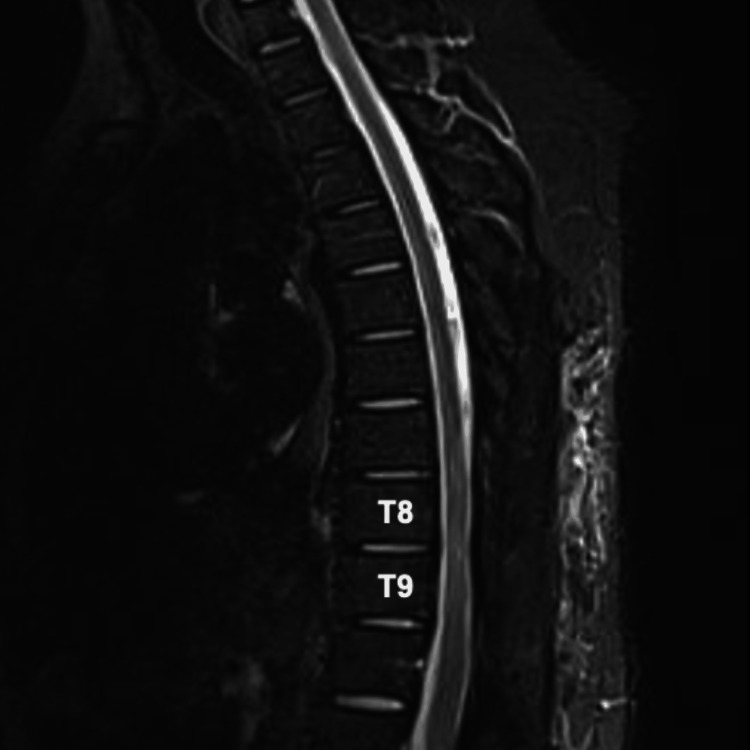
Postoperative T2 weighted sagittal MRI image demonstrating amelioration of spinal cord compression, epidural lesion, and spinal cord edema at the T8 and T9 levels. T8: thoracic vertebral segment 8; T9: thoracic vertebral segment 9
